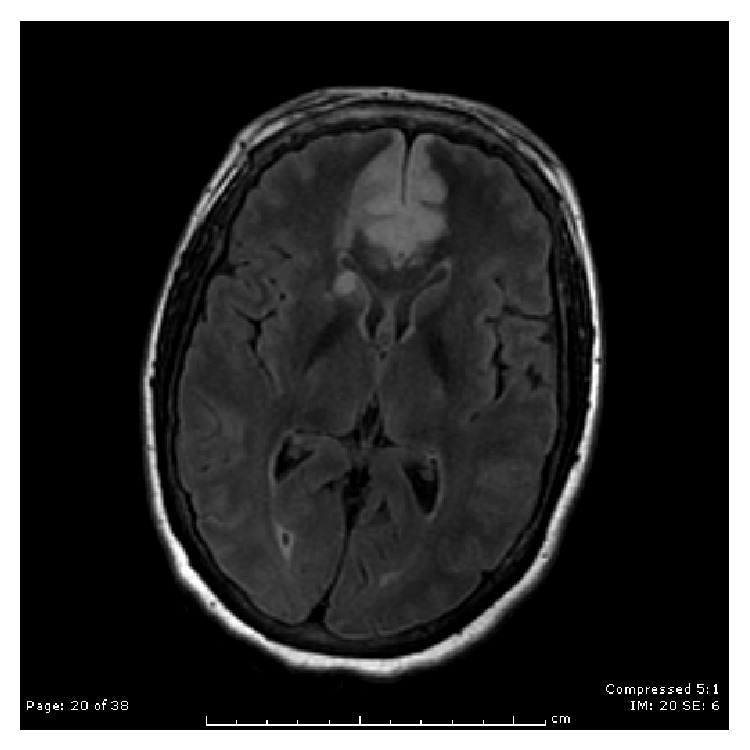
FLAIR MRI showing hyperintense lesion bilateral ACA territory and head of caudate on right side.
